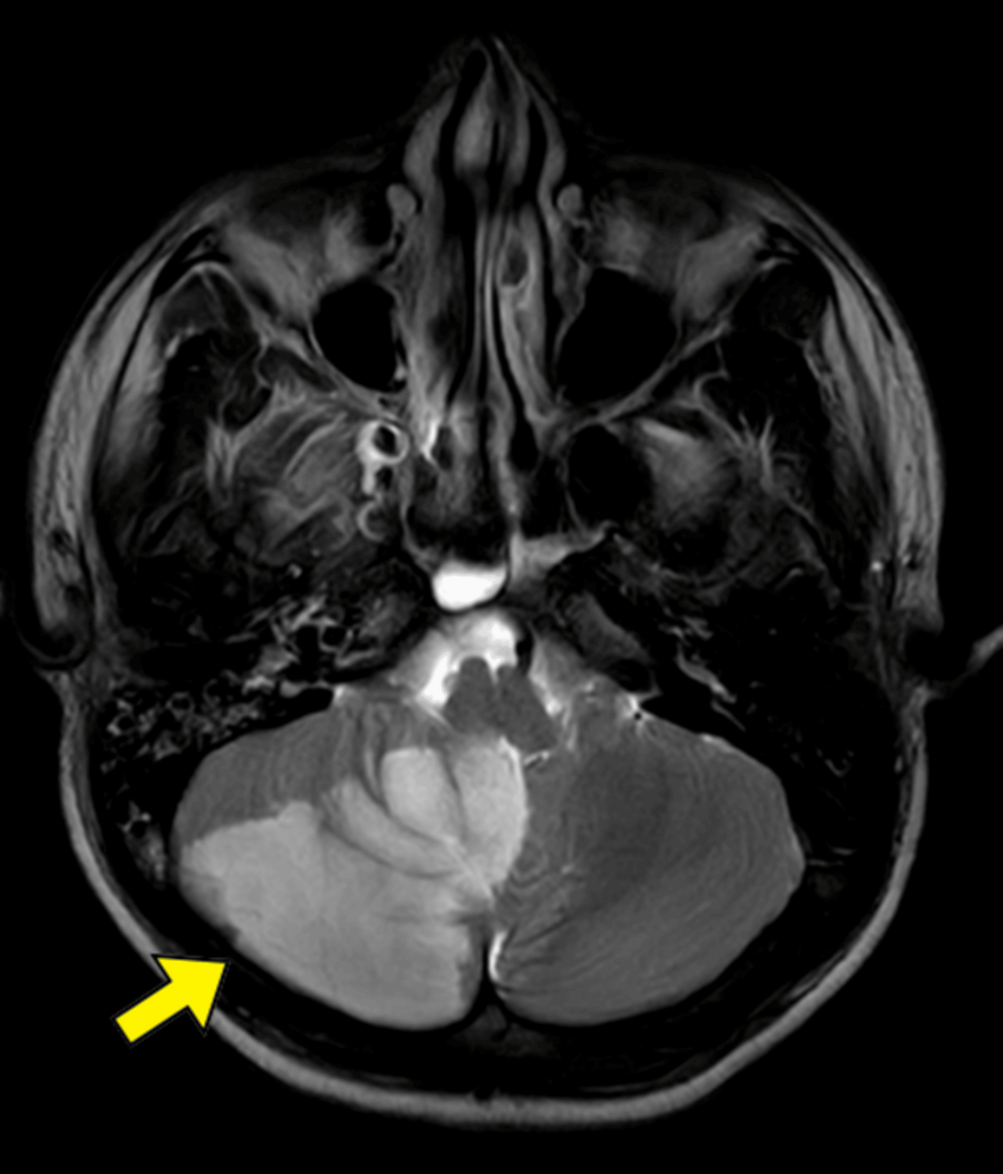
Magnetic resonance imaging (MRI) of the brain showing extensive area of hyperintensity in the right cerebellar hemisphere and mid-brain (yellow arrow).
